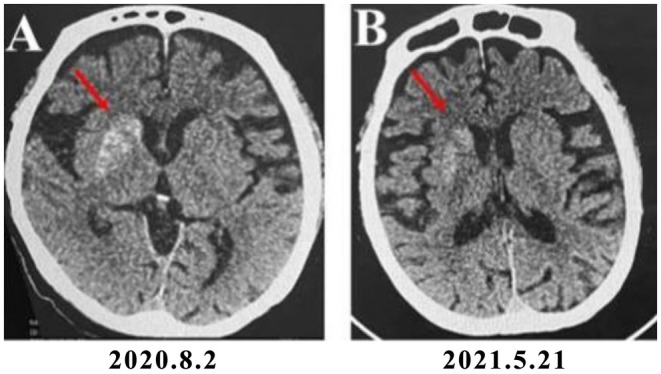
Serial non‐contrast axial head CT scans. (A) The initial scan from August 2, 2020, demonstrates a subtle hyperdensity in the region of the right caudate nucleus and putamen (red arrow), consistent with diabetic striatopathy. (B) A follow‐up scan performed on May 21, 2021, shows a marked reduction in the size and density of the lesion (red arrow), correlating with clinical improvement.
